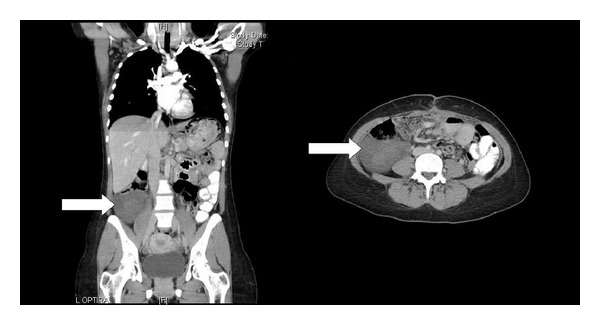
Frontal (*left panel*) and transverse (*right panel*) views of the CT scan performed postoperatively. A well-defined oval hypodense uniform mass is appreciated at the right iliac fossa measuring 10 × 8.4 cm with a central rounded higher density soft tissue area within it (white arrows).
